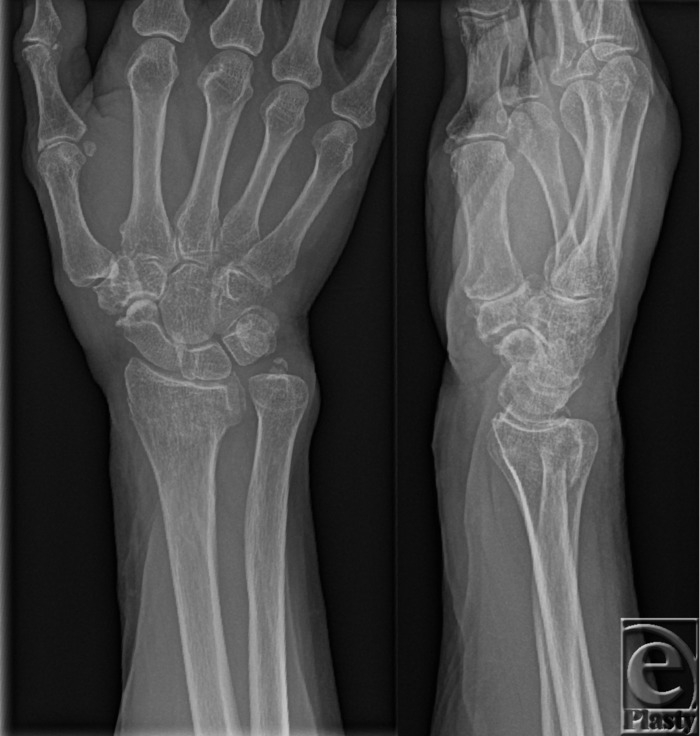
Anteroposterior and lateral radiographs of the right wrist showing a Frykman II displaced distal radius fracture and ulnar styloid avulsion fracture.
